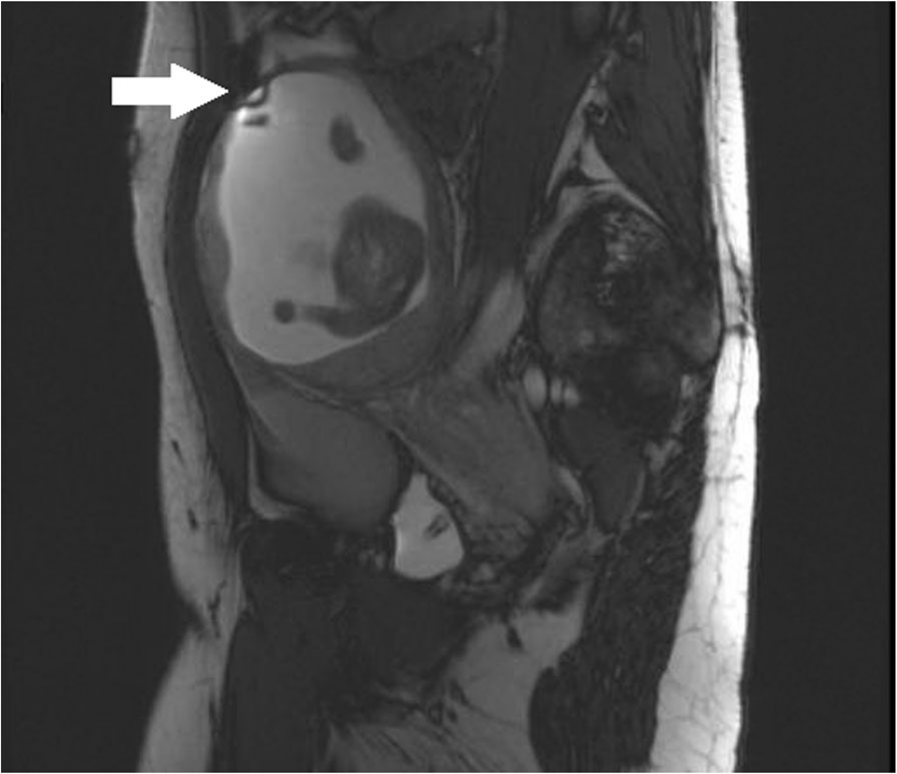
T2-weighted pelvic MRI at 17 weeks gestational age, with thin left uterine horn myometrial thickness (indicated with a white arrow), with a known amniotic band
